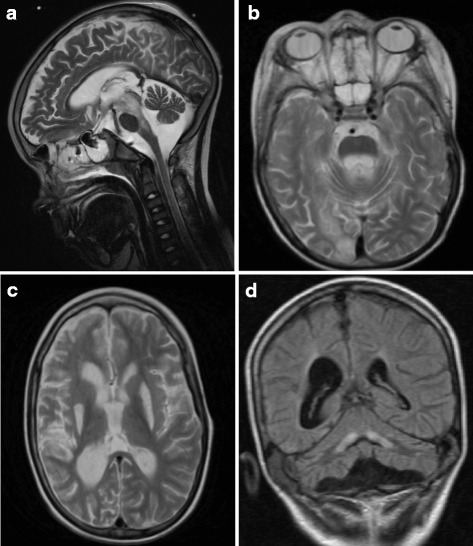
MRI findings of proband 1. a, b: sagittal and axial T2-weighted sections showing the presence of bilateral hyperintense alterations in the mesencephalon, pons and subthalamic nuclei, all markedly swollen; c: axial T2-weighted image showing bilateral necrosis of putamina and left caudate nuclei; d: coronal T1-weighted image showing cerebellar atrophy, dentate nuclei hyperintensity and ventricular enlargement
